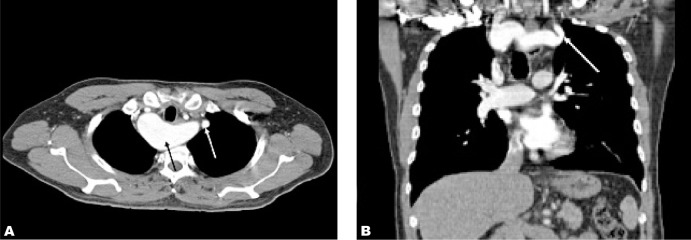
A. Axial CT image of the right-sided aortic arch in the same patient. Kommerell’s diverticulum (black arrow) and origin of aberrant left subclavian artery (white arrow) is seen in this image. B. Coronal multiplanar reformatted CT image of the origin of aberrant left subclavian artery (white arrow).
